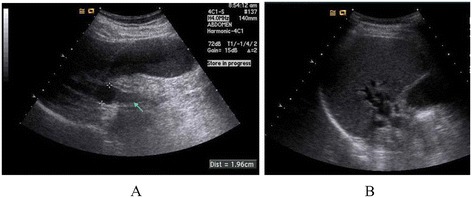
Abdominal ultrasound. (A) A tubular mass in distal common bile duct (arrow) and distended gallbladder and common hepatic duct. (B) Dilated intrahepatic bile ducts.
